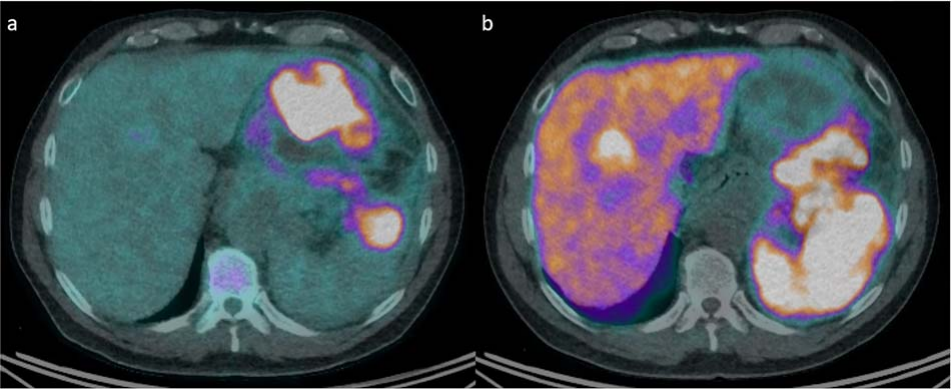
FDG and DOTATATE PET/CT of the mass. (a) Intensely FDG-avid mass with patchy regions of relative photopenia and necrosis. (b) Heterogenic DOTATATE uptake with poor uptake in the FDG avid components at the inferior aspect of the pancreatic mass and superior-posteriorly to the stomach.
